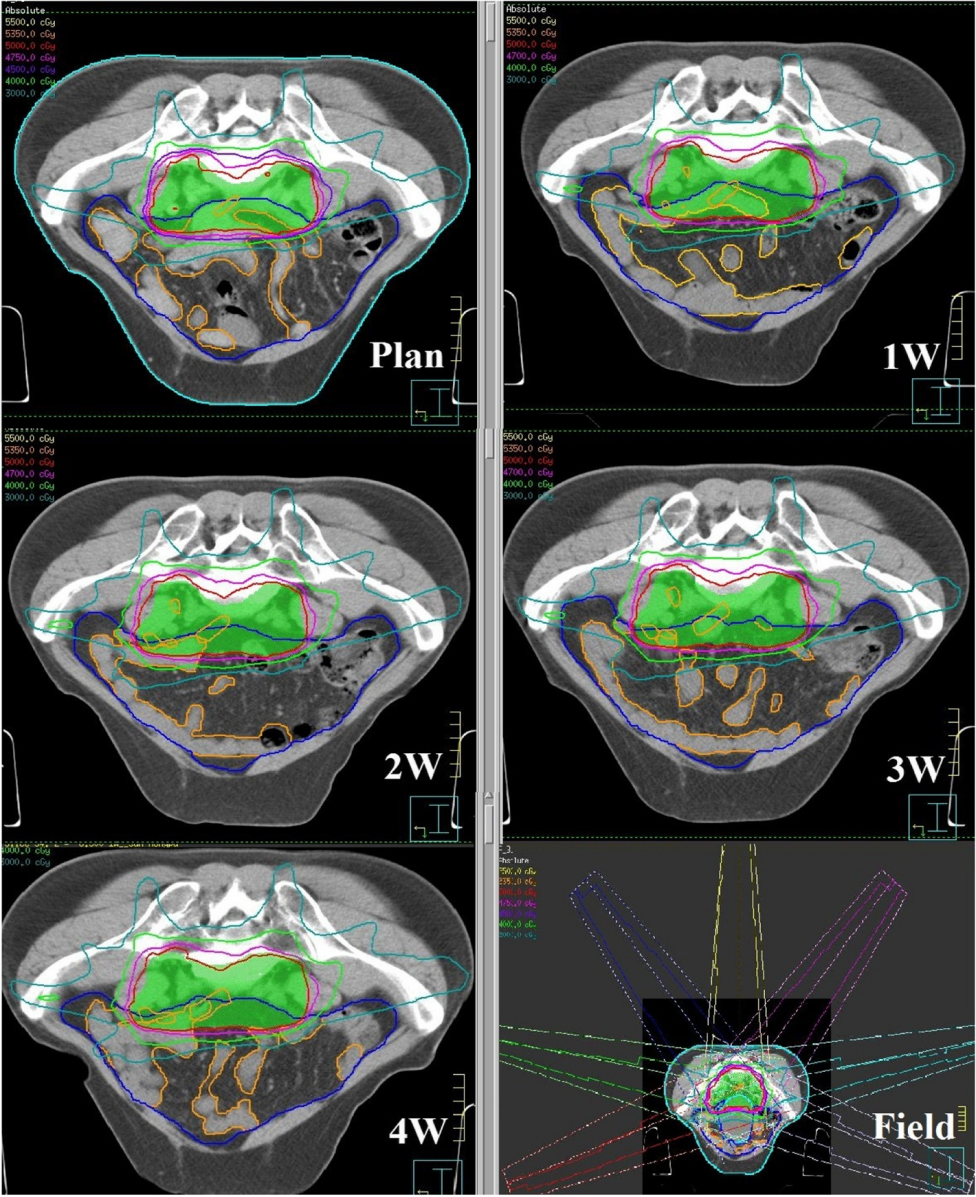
An example of a rectal cancer patient’s PS and BL contours and dose distribution based on different CT scans during treatment. The green, blue, and orange contours represent PTV, PS, and BL, respectively. The innermost and outermost dose lines are 50 Gy and 30 Gy, respectively. Picture Plan, 1 W, 2 W, 3 W, 4 W show the variations of PS and BL’s contours and dose distribution during treatment, picture Field show the radiotherapy field setup
